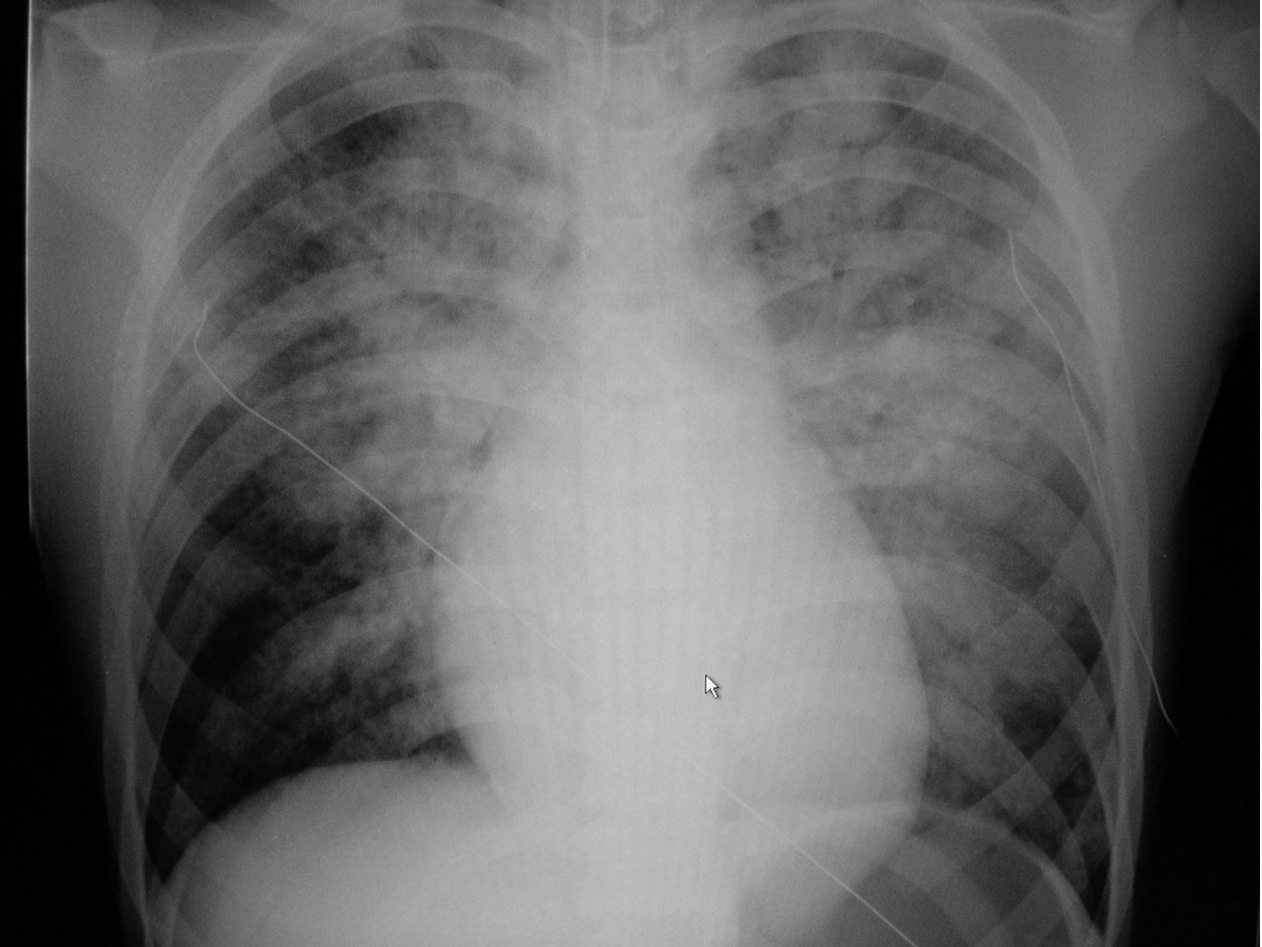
Chest x-ray showed bilateral acute pulmonary edema.
